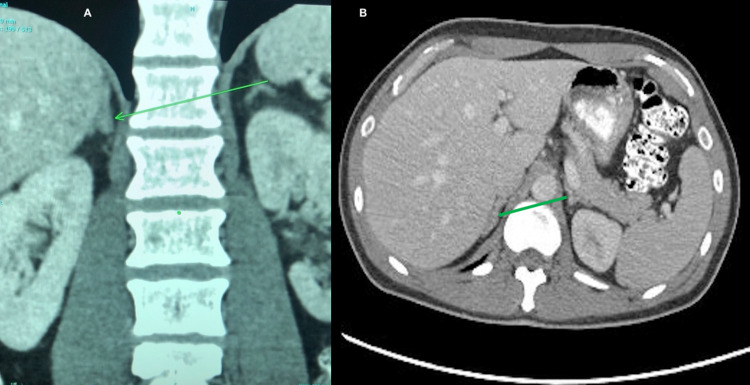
CT scan of the abdomen: (A) coronal section, (B) axial section showing asymmetric thickening of the medial lobe of the right adrenal gland
